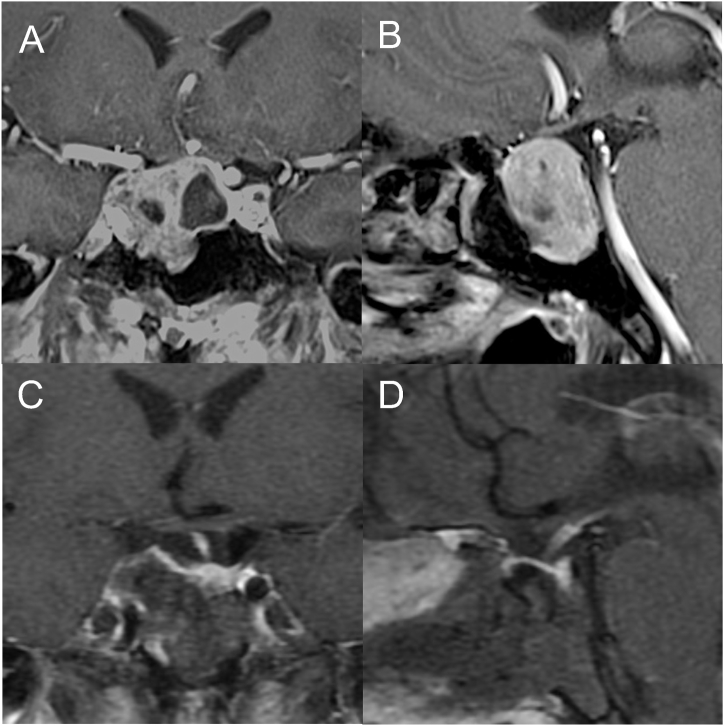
Representative Case 1: A 44-year-old man with GH-producing pituitary neuroendocrine tumor. The preoperative values were as high as 25.6 ng/mL for GH, 86.3 ng/mL for prolactin, and 805 ng/mL for IGF-1 (standard range: 92–255 ng/mL). Preoperative coronal (A) and sagittal (B) T1-weighted MR images after injection of gadolinium showing a tumor with maximum diameter of 29.0 mm and lateral extension of Knosp grade 3A to the right. The normal pituitary gland was on the left side. Postoperative coronal (C) and sagittal (D) T1-weighted MR images after injection of gadolinium showing complete removal of the tumor. Postoperative GH level was 0.72 ng/mL, 3.2 ng/mL for prolactin, and IGF-1 level was 239 ng/mL, showing remission without affect on other pituitary hormones after surgery.
